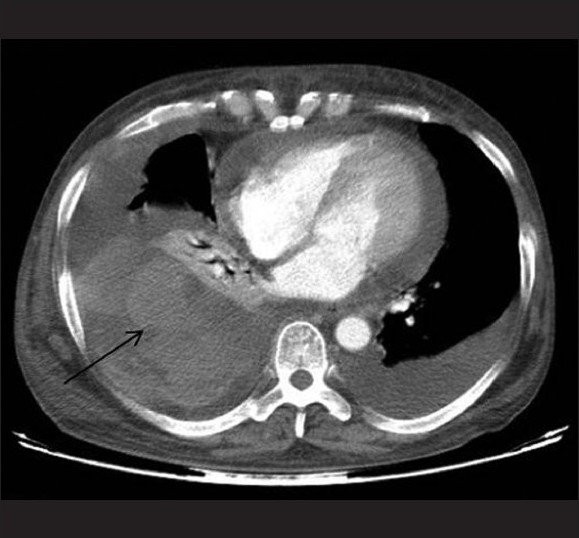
Computed tomography scan of chest shows right-sided hemothorax, left pleural effusion, and pericardial effusion
